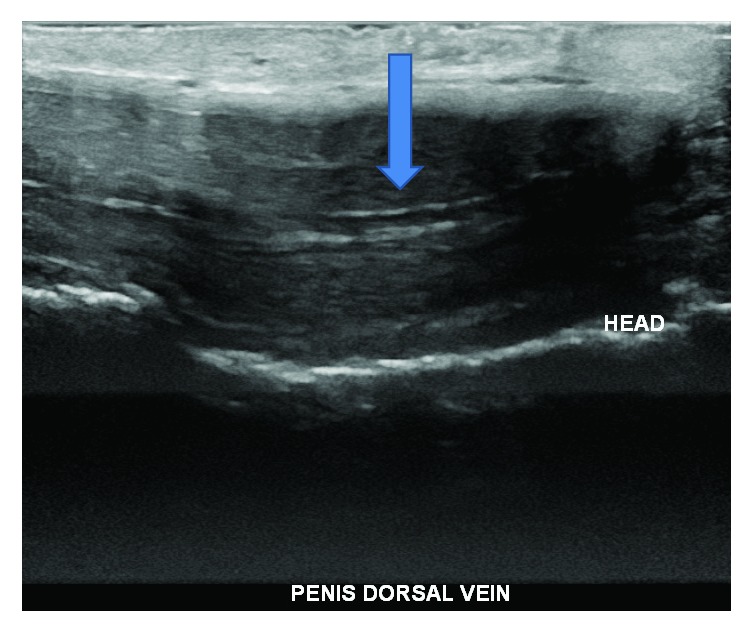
Penile ultrasound with the arrow directed towards thrombus in the dorsal vein. It is noncompressible.
